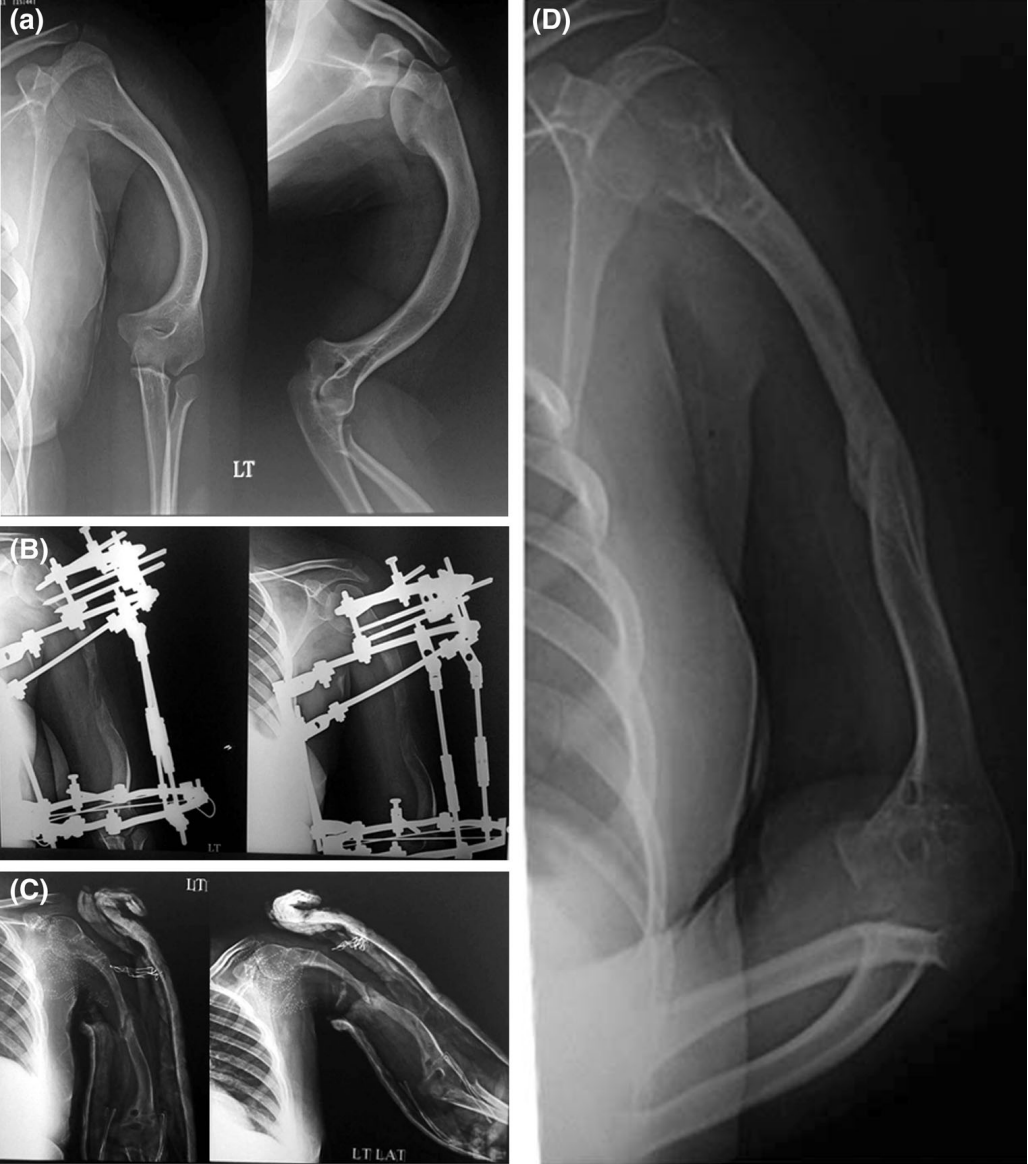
a A 14-year-old patient with humeral shortening and deformity. b X-ray at the end of lengthening and deformity correction. c Fracture of the regenerate after fixator removal with the arm in a splint. d Follow-up X-rayInferior subluxation of the shoulder developed during treatment in two cases and resolved with physiotherapy.Premature consolidation of the regenerate in one case. The treatment was continuation of distraction at a rate of 1.5 mm/day which led to accumulation of the force of distraction and, finally to forcible wide separation of the osteotomy site with severe pain. The gap was then closed acutely to reduce the pain, a waiting period for 3 days followed by distraction at a rate of 1 mm/day.
